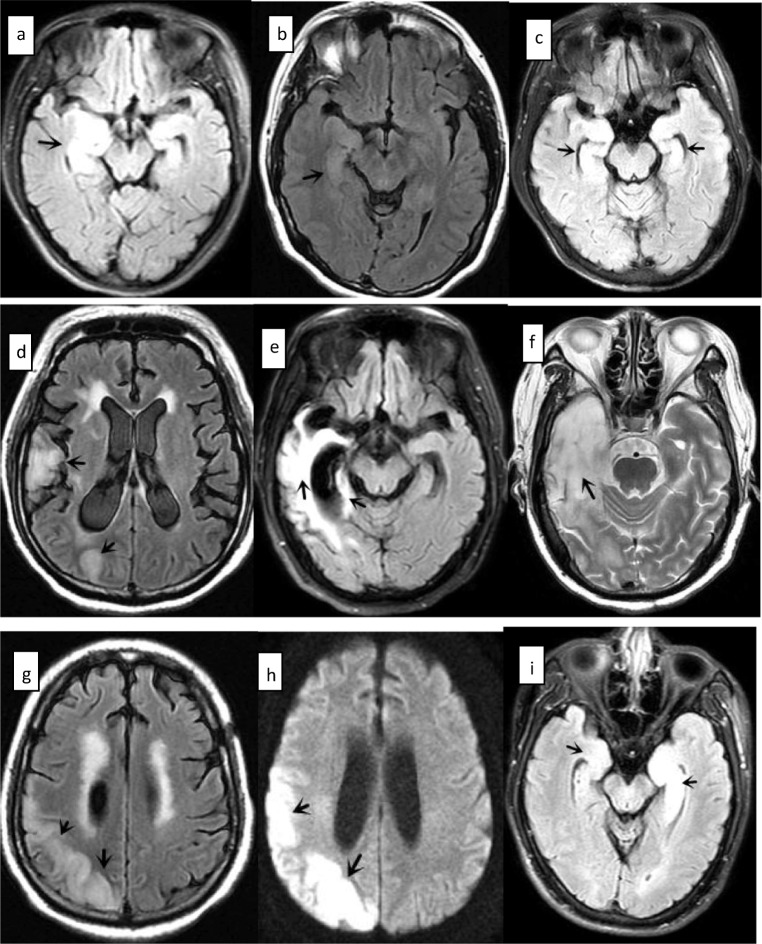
Patient 1: a T2-FLAIR showing hyperintensity in the right hippocampus, parahippocampal gyrus, and amygdala. Patient 2: b T2-FLAIR showing hyperintense lesions in the right temporal lobe. Patient 3: c T2-FLAIR showed hyperintensities in the hippocampus, bilaterally, and in the medial temporal lobe. Patient 5: d T2-FLAIR showing abnormal signals in the right temporal lobe. e T2-FLAIR showing abnormal signals in the right temporal lobe and hippocampus. f T2-weighted sequence showing hyperintense lesions in the right temporal lobe. g T2-FLAIR showing hyperintensity in the right temporal occipital lobes. h Diffusion-weighted imaging sequence showing hyperintensity in the right temporal and occipital lobes. The lesions described above are marked with arrows. Patient 7: i T2-FLAIR showing abnormal signal shadows in the hippocampus, bilaterally (more obvious on the left). Patient 4 and patient 6 did not show any encephalitis lesions on magnetic resonance imaging. FLAIR: fluid-attenuated inversion recovery
